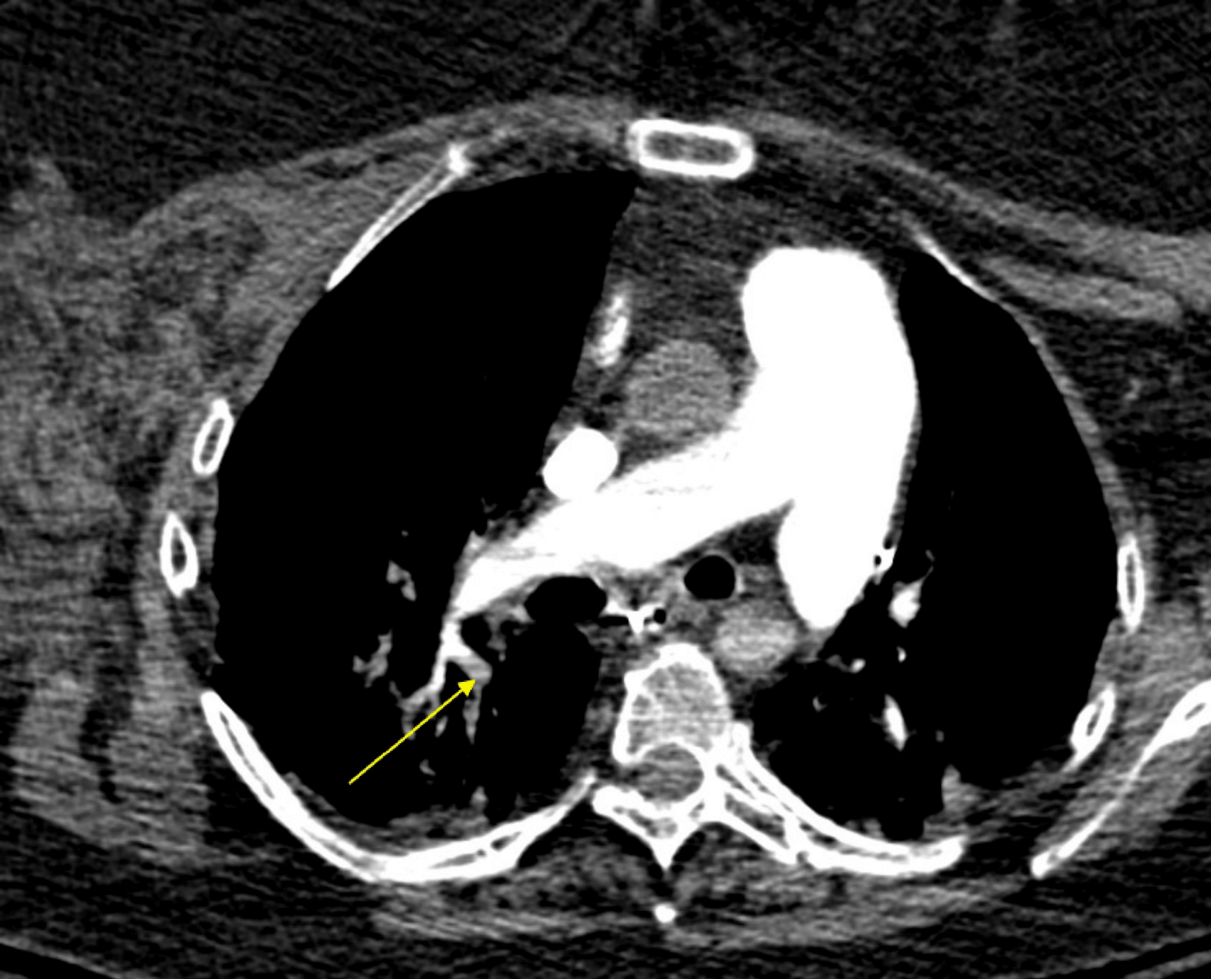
CT angiography chest on hospital day three demonstrating pulmonary emboli in the right lower lobe segmental branches (yellow arrow).
